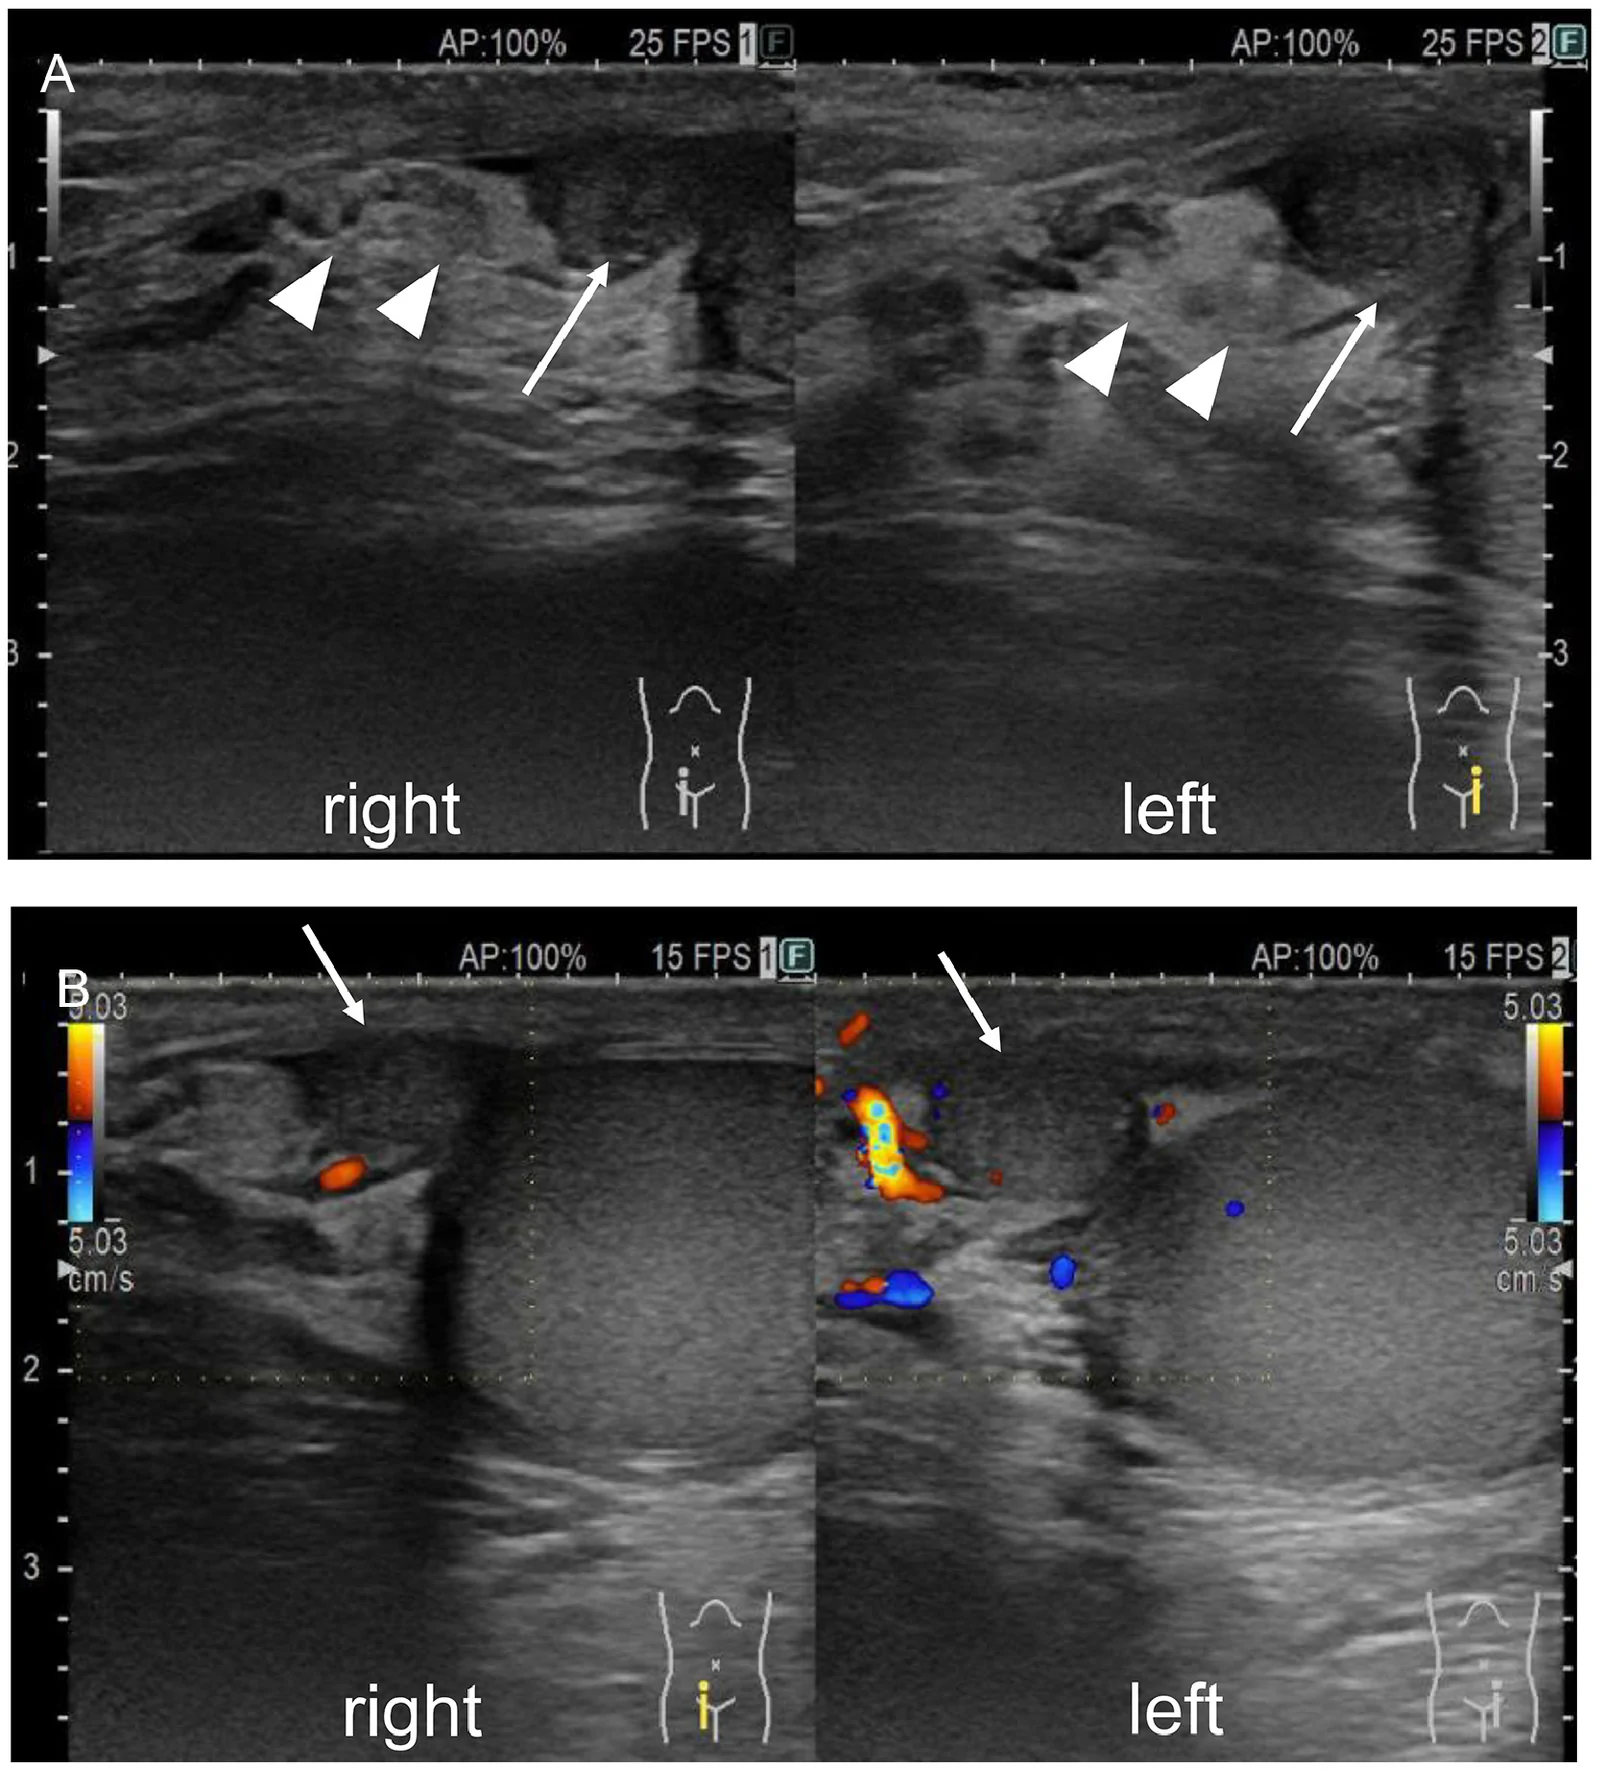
Scrotal ultrasound performed by a urologist; the right and left sides are labelled on the images. (A) B-mode image shows swelling of the spermatic cords (arrowheads) and the epididymides (arrows, 1 on each side). (B) Color Doppler image shows increased vascularity of the epididymides bilaterally (arrows, 1 on each side).
